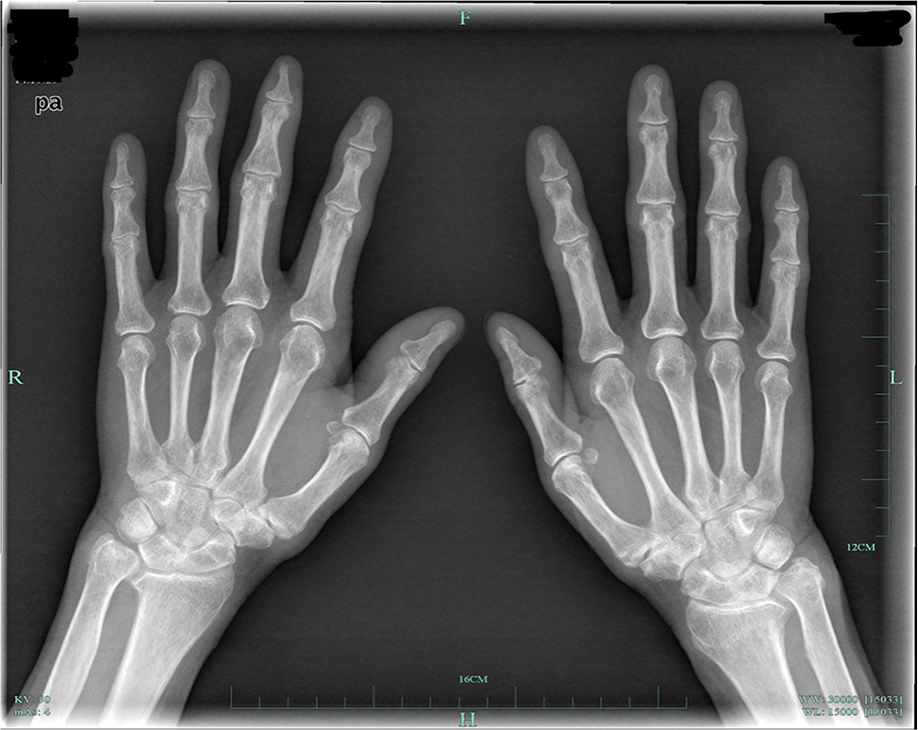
Plain X-ray films document no tumor recurrence in three years after surgery
